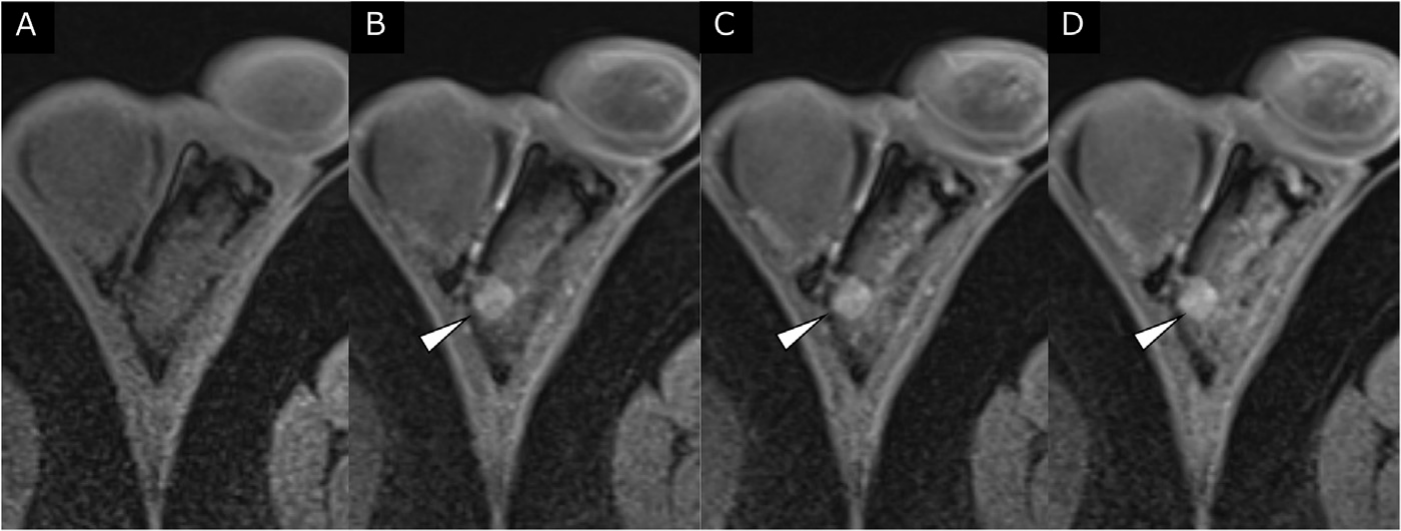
Dynamic contrast-enhanced MRI, axial images. (A) Precontrast fat-suppressed T1WI failed to demonstrate the tumor. The mass showed inhomogeneous contrast enhancement on postcontrast scans at (B) 40 seconds, (C) 70 seconds, and (D) 150 seconds after intravenous gadolinium injection.
